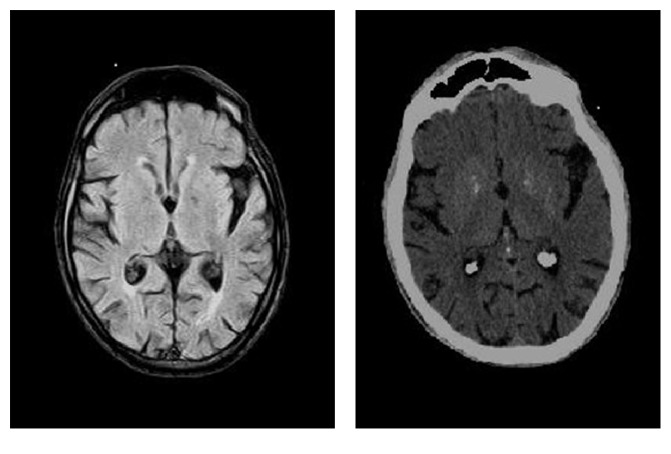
MRI scan on the left and CT scan on the right performed under pharmacological sedation in order to reduce motion artifacts, showing moderate diffuse cortical atrophy and small bilateral basal ganglia calcifications.
